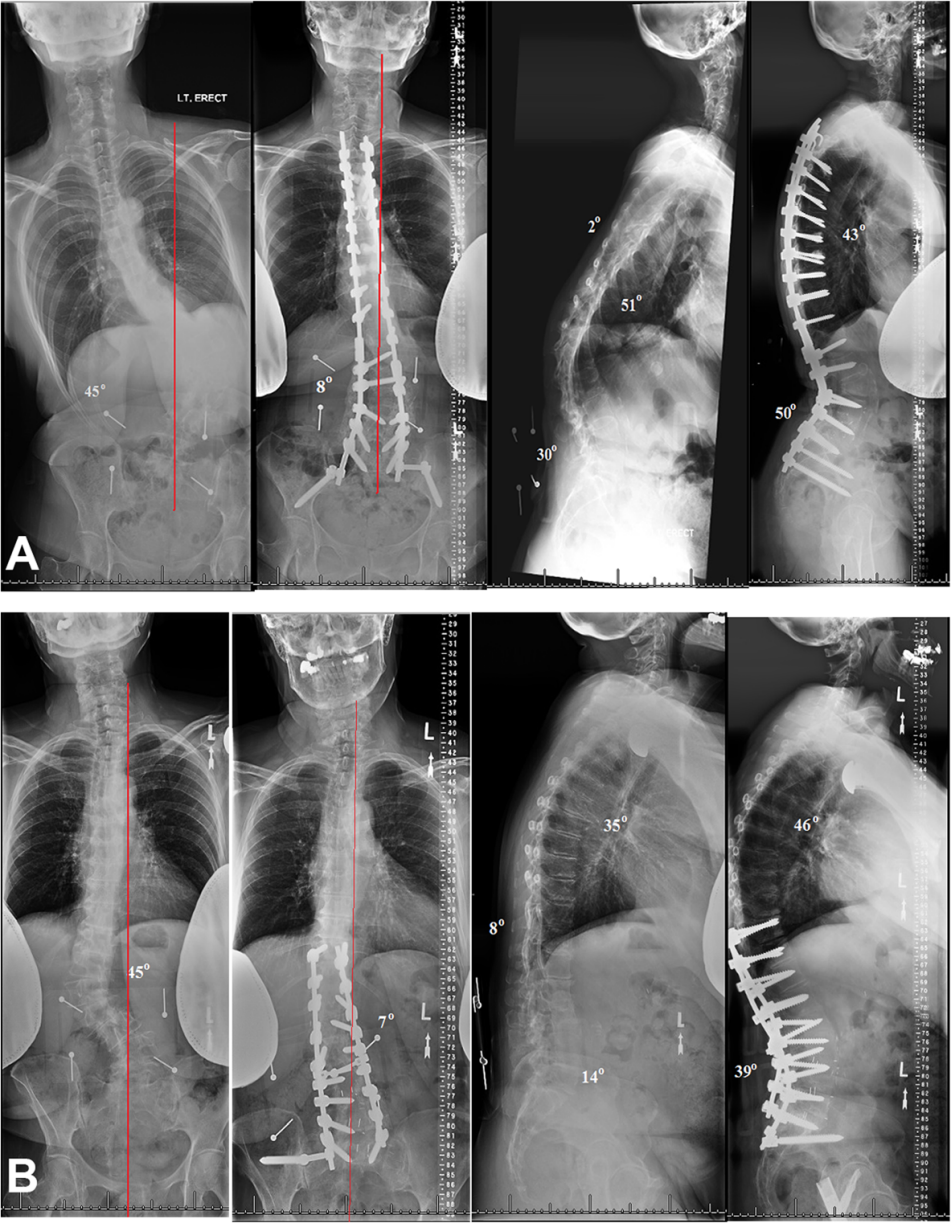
Two 63- and 79-year-old women suffering from adult spinal deformity underwent 3-column osteotomy (A) and standard surgical management (B), respectively. Three years after surgery, anteroposterior and lateral radiographs demonstrate marked correction, satisfactory alignment, and a solid spinal fusion.
